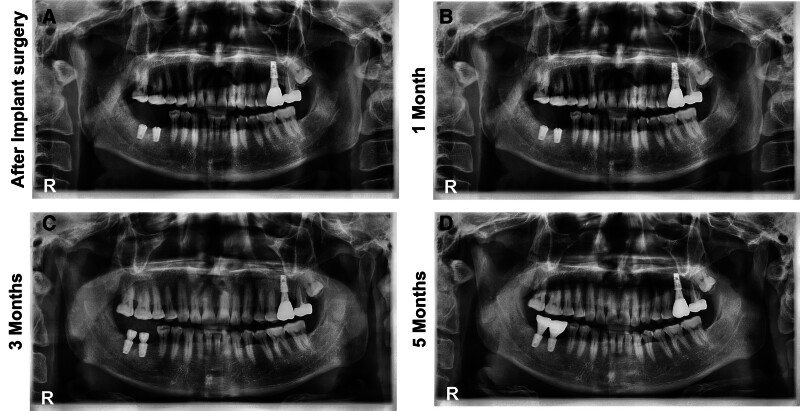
Panoramic view series following dental implant. (A) Following the removal of the osteochondroma, dental implant surgery on #46 and #47 regions with surgical extraction of #18 and #48, was performed under local anesthesia. (B) One month post-dental implantation. The patient was instructed to wear the occlusal splint at least 8 hours a day. (C) Three months post-dental implantation, a secondary implant surgery to reveal the fixture was performed and healing abutments were placed. (D) Five months post-dental implantation, the final prostheses for the right mandibular molars were delivered. CT = computed tomography.
